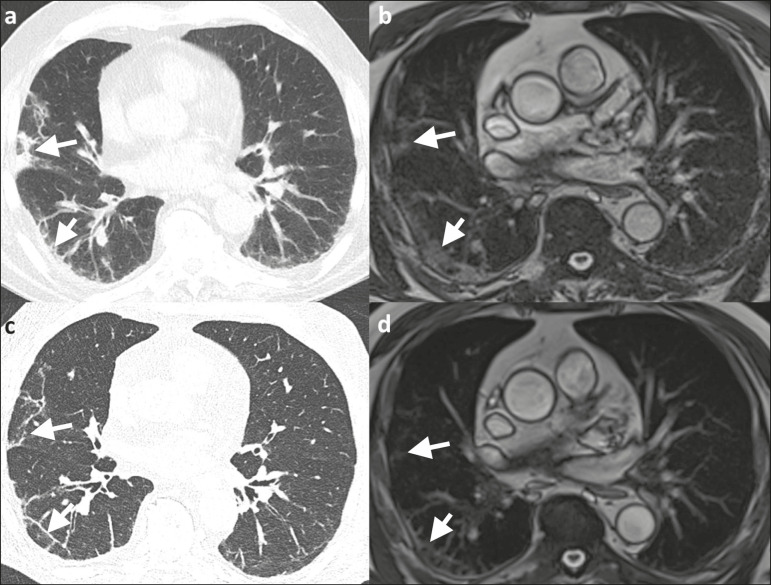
Appearance of pulmonary changes in COVID-19 pneumonia on 1.5 T TrueFISP MRI over time. Chest CT (a) of a patient who was diagnosed with COVID-19 via RT-PCR 28 days earlier, showing patchy and reticular consolidations in the middle lobe and right lower lobe (arrows). The opacities are almost equally as visible on an MRI scan (b) performed 8 days later. Follow-up CT scan (c) and follow-up MRI scan (d) acquired 56 and 59 days, respectively, after the RT-PCR almost equally show the reduction of the opacities to linear residuals (arrows).
